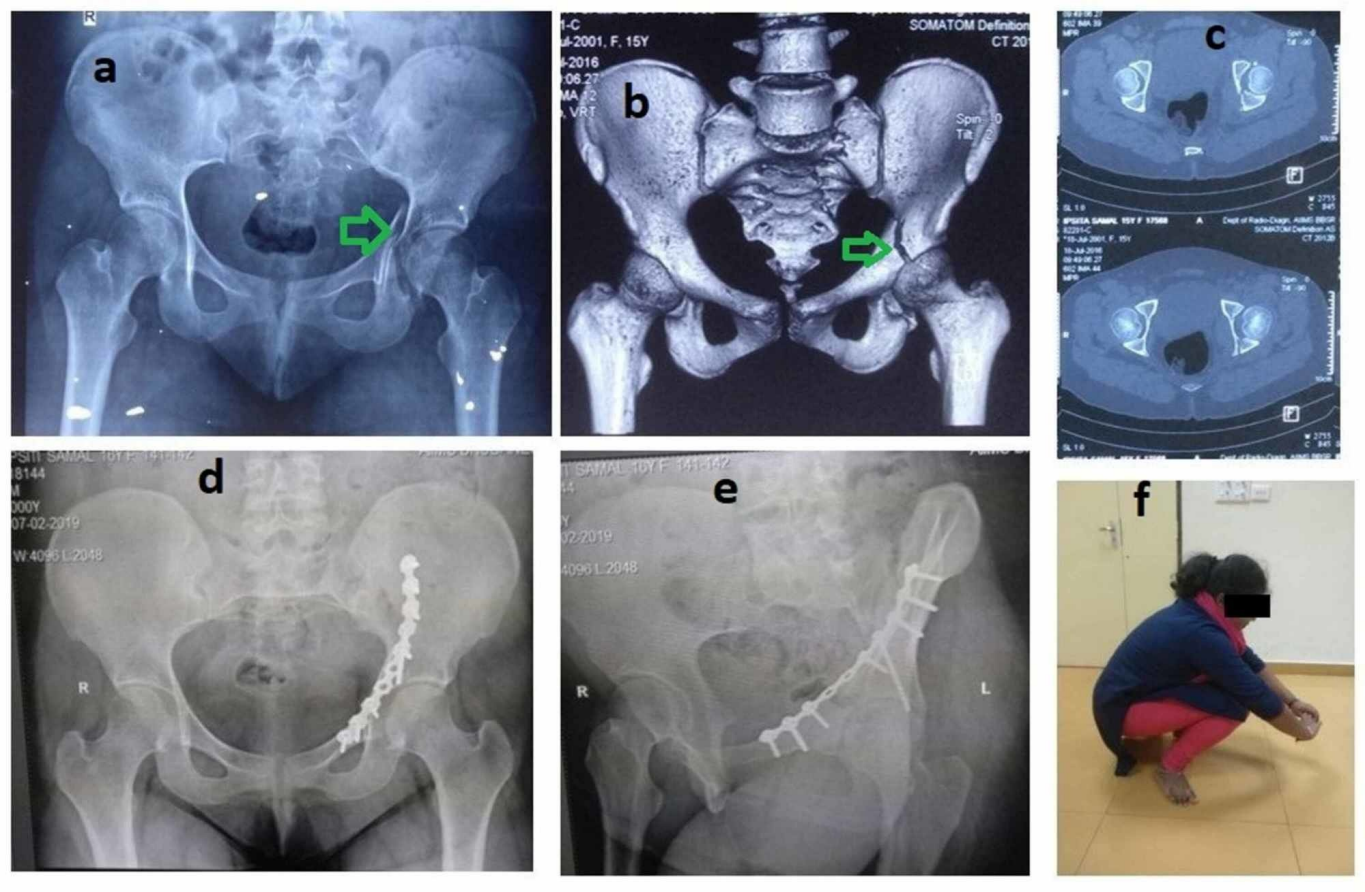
A case of transverse fracture (green arrows) with final postoperative X-rays and functional outcome a: preoperative radiograph; b, c: preoperative CT; d, e: two-year follow-up radiograph; f: clinical photograph of the patient squatting CT: computed tomography
